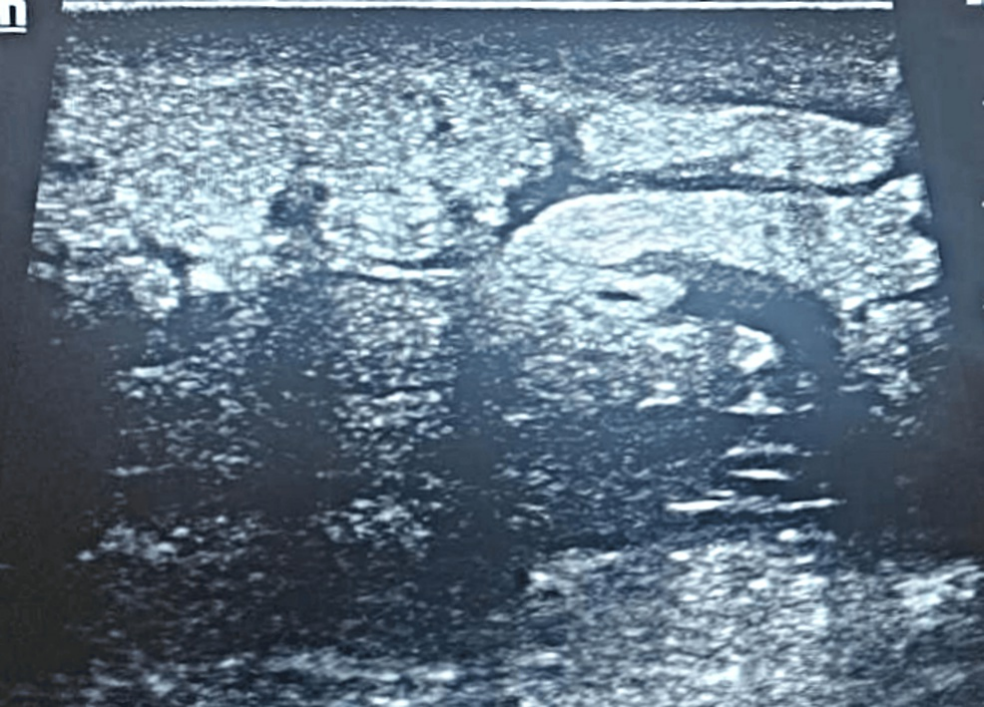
Emergency ultrasound image showing soft tissue infiltration.
